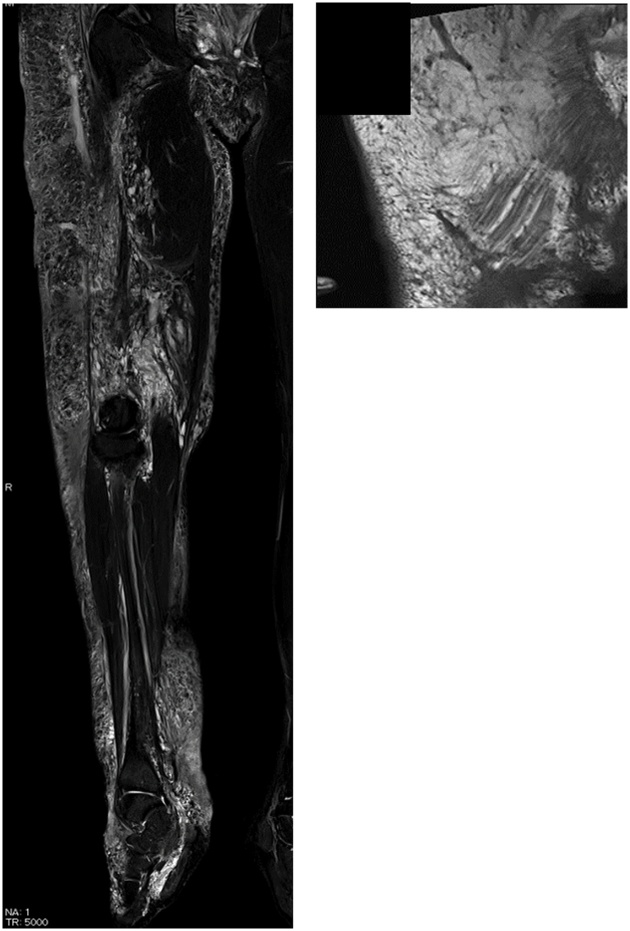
Vascular malformation was detected in the whole right leg and buttocks via MRI at the initial diagnosis. The mixed vascular malformations were noted in a thigh and the buttocks with skin lesions in particular.
